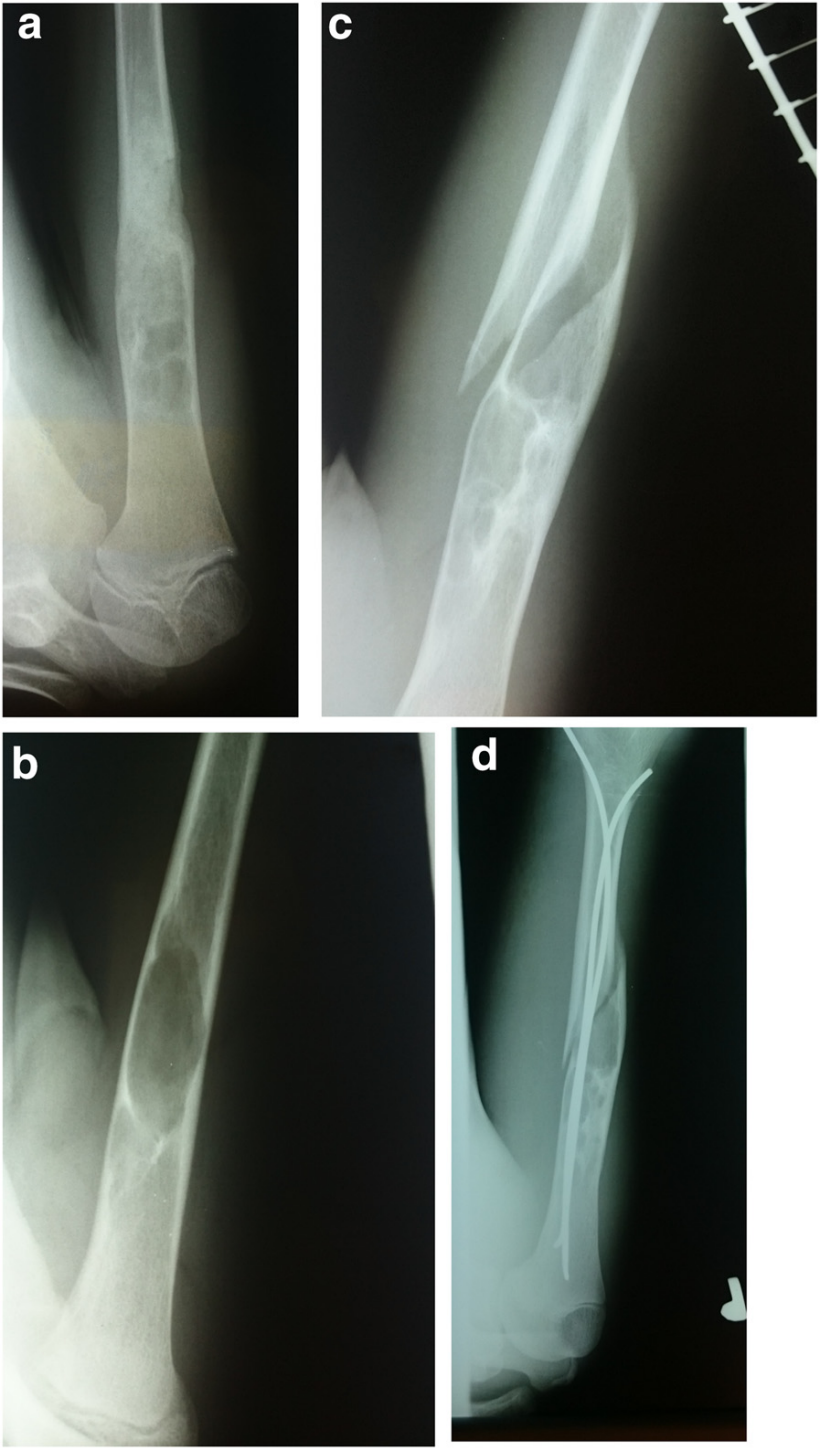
Example of a patient with SBC with recurrence after curettage and bone grafting treated with titanium elastic nails. a X-ray of the lesion at 3 months postoperatively; (b) at 12 months postoperatively; (c) recurrence with pathological fracture; (d) X-ray follow-up directly after the titanium elastic nails implantation
